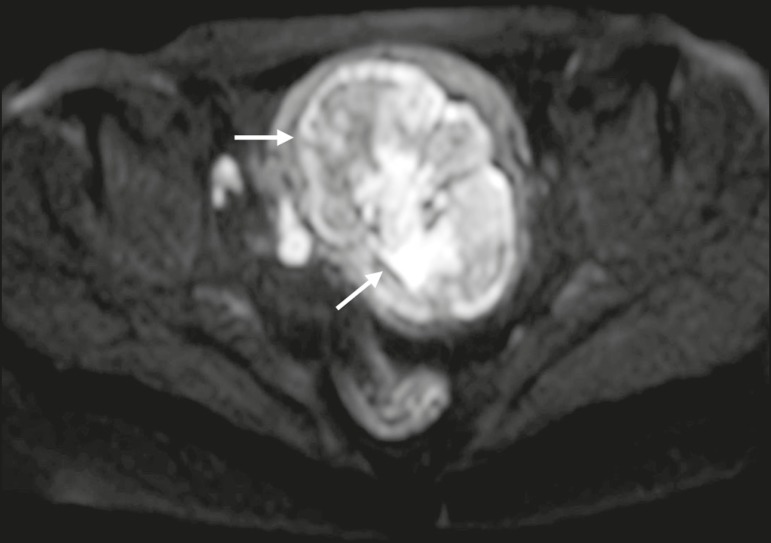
MRI of a female patient with leiomyosarcoma. Axial DWI at b = 1000
